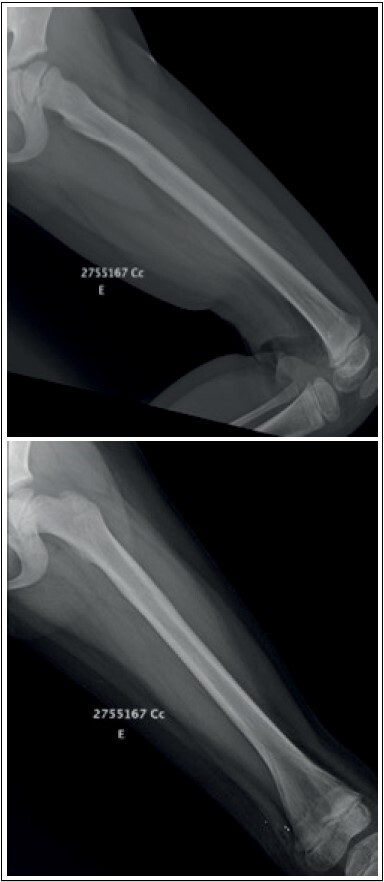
Radiography after nailing removal (12 months after surgery). Same patient as Figure 2 .
